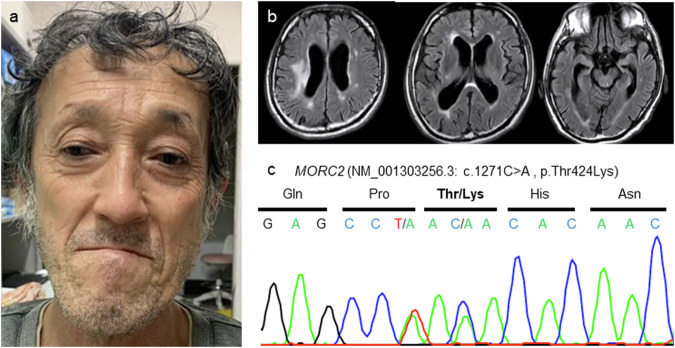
The patient's facial features, brain MR image, and sequencing analysis of *MORC2.* a Facial features of the patient. The patient presented with a long face, narrow jaw, and thin upper lip. b Brain MR image of the patient. MRI revealed slightly atrophied cortices and hippocampi, enlarged lateral ventricles, and high signals in the right corona radiata and deep cerebral white matter on FLAIR imaging. c Sequencing analysis of *MORC2* in the patient. Exome sequencing revealed a novel heterozygous variant in *MORC2* (NM_001303256.3: c.1271 C > A, p.Thr424Lys).
